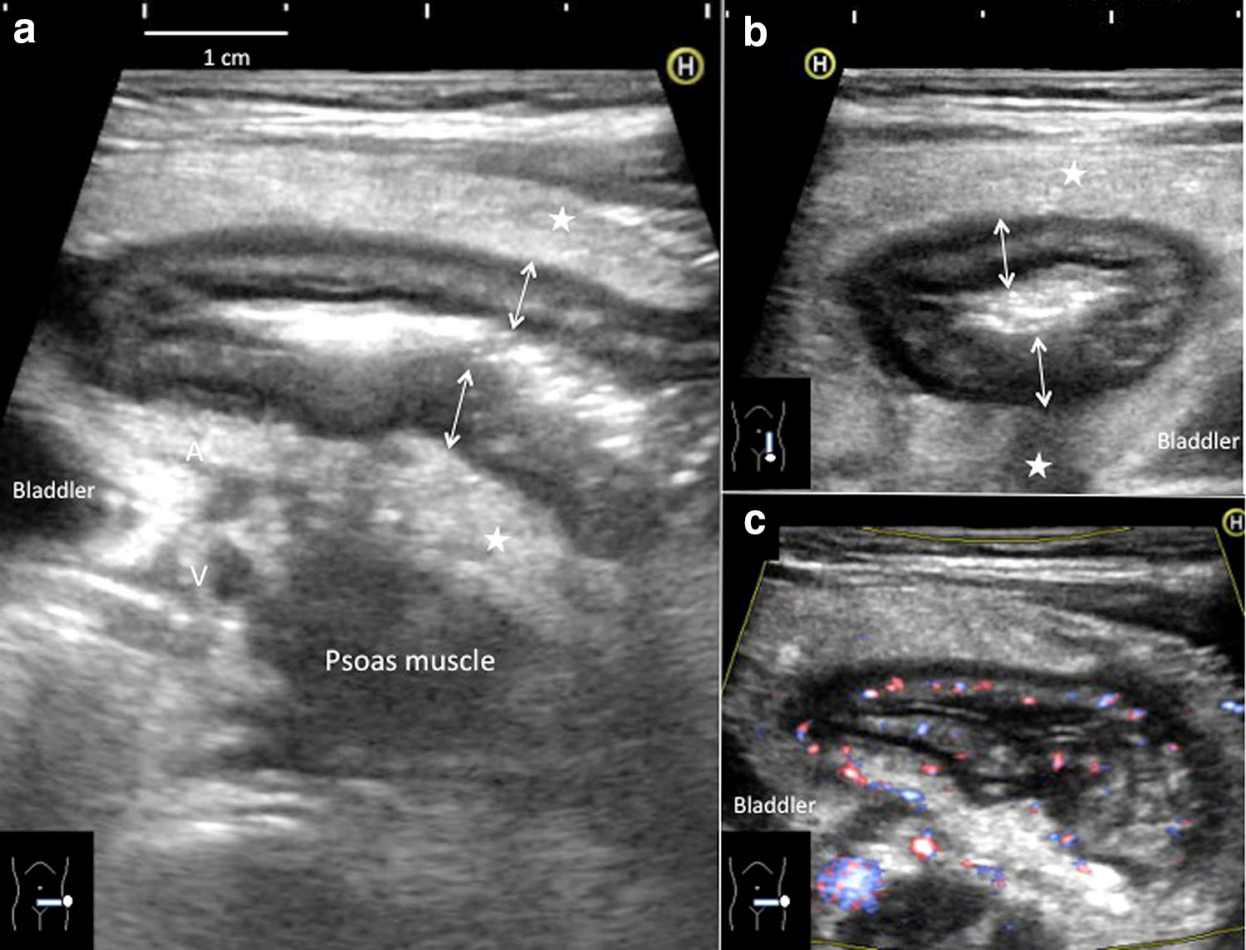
Abdominal ultrasonography on admission. a
